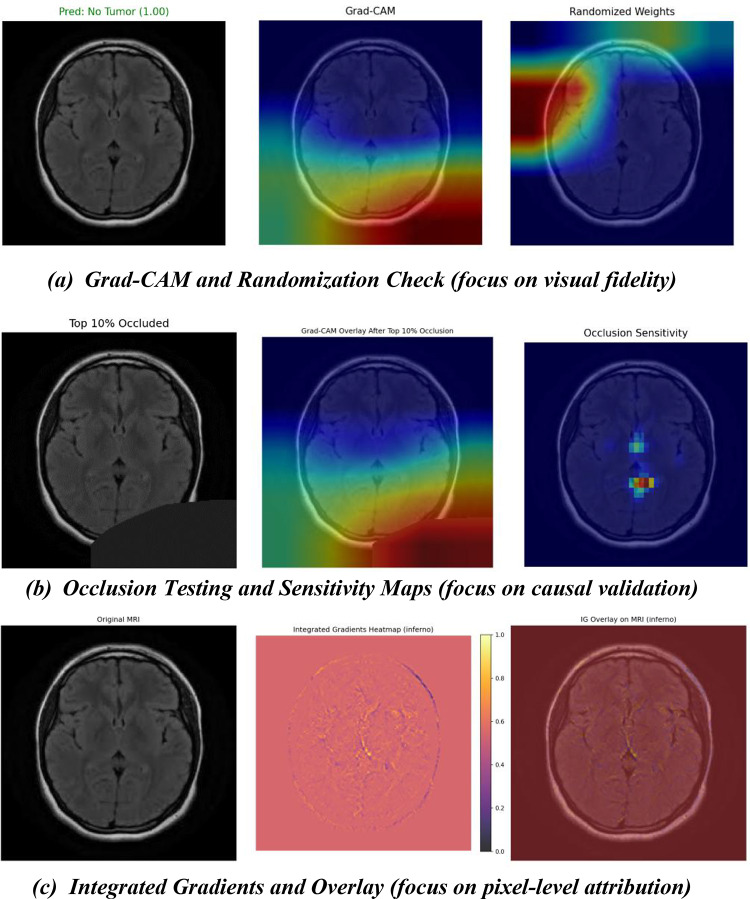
Representative Example of XAI Methods on Brain MRI Non-Tumor Case from External Dataset (a) Grad-CAM highlights tumor regions, while randomization disrupts activations. (b) Occlusion testing and sensitivity maps confirm causal relevance of highlighted areas. (c) Integrated Gradients overlays, visualized with a perceptually uniform colormap (Inferno), provide pixel-level attribution consistent with Grad-CAM, reinforcing interpretability reliability.
